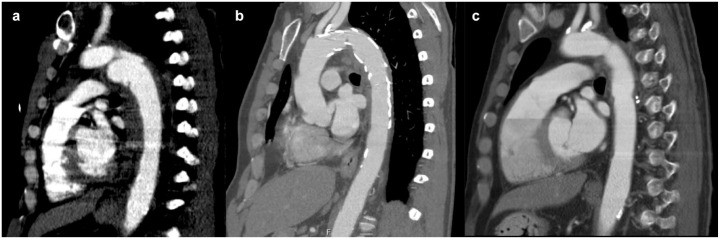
Sagittal computed tomography scans demonstrating (a) grade 3 blunt thoracic aortic
